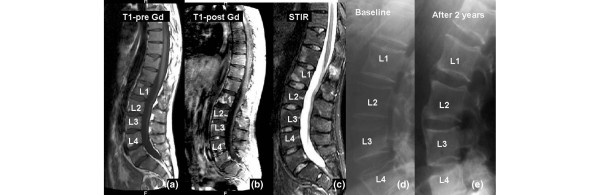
Formation of new syndesmophytes in the upper and lower edges of L1/L2 and L2/L3. (a) T1-pre-gadolinium (T1-pre-Gd) image. Spinal inflammation in the same area is assessed by both magnetic resonance imaging (MRI) sequences: (b) T1-post-gadolinium (T1-post-Gd) and (c) short-tau-inversion-recovery (STIR). Inflammation at baseline is seen as a 'spot' in the T1-post-Gd image only after application of gadolinium. The STIR image shows signs of inflammatory activity in the same vertebral regions. Formation of new syndesmophytes in the upper and lower edges of L1/L2 and L2/L3 is seen in conventional x-rays developing from (d) baseline to (e) 2 years later.
